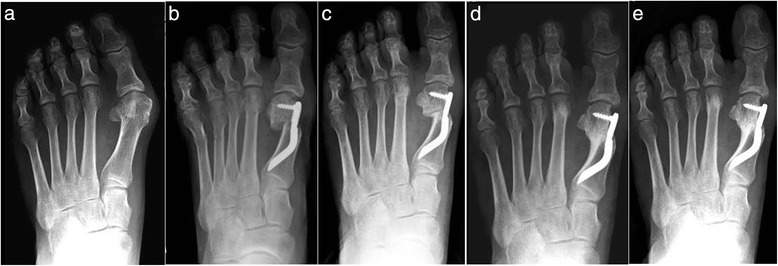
Case 6. A 54-year-old woman with moderate HV; antero-posterior radiographic images at: a Preoperative period. b 1-month follow-up. c 3-month follow-up. d 6-month follow-up. e 48-month follow-up showing the maintained correction of the deformity
